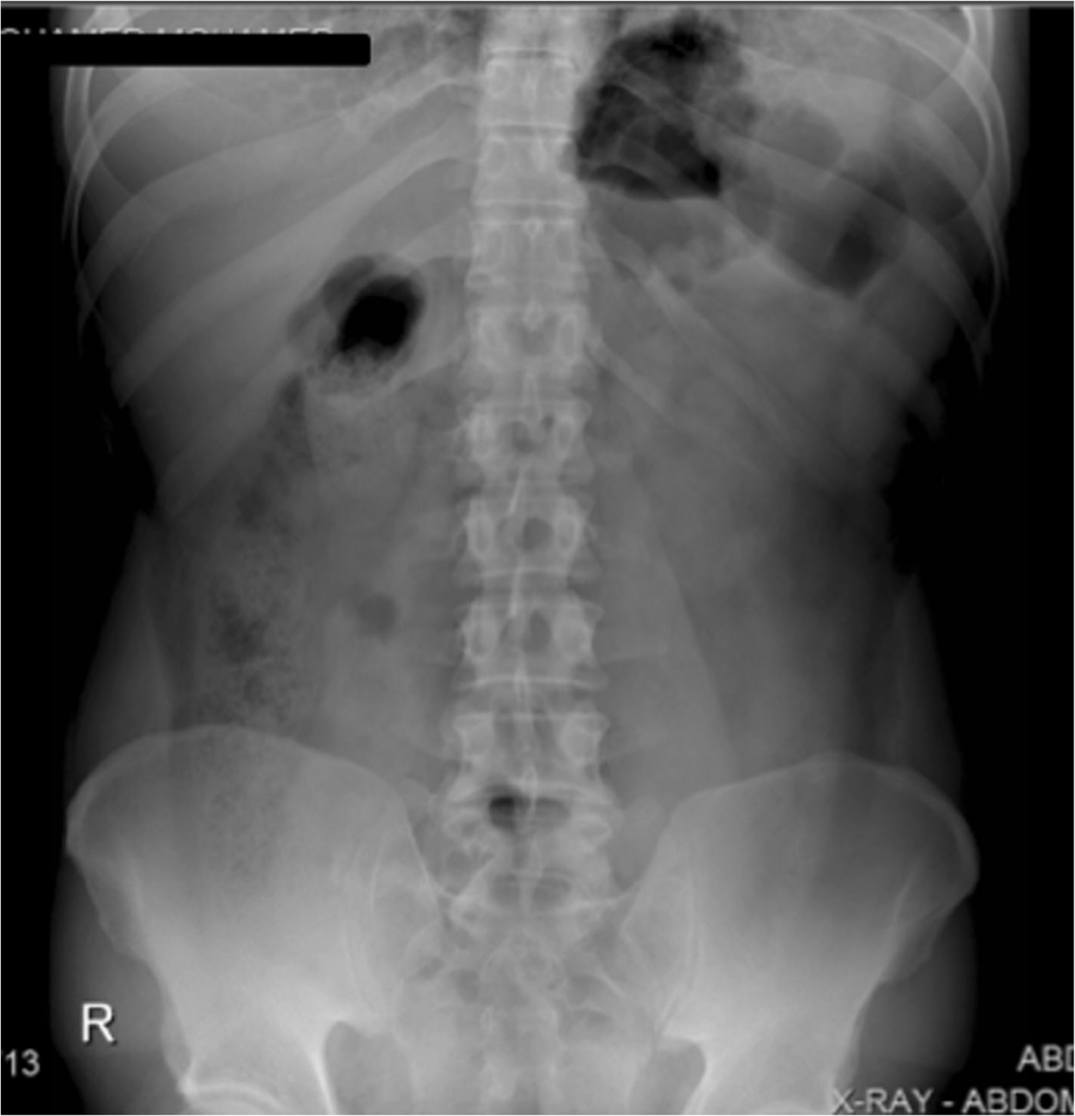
An abdominal Radiograph showed no intraperitoneal free air, otherwise an unremarkable x-ray
